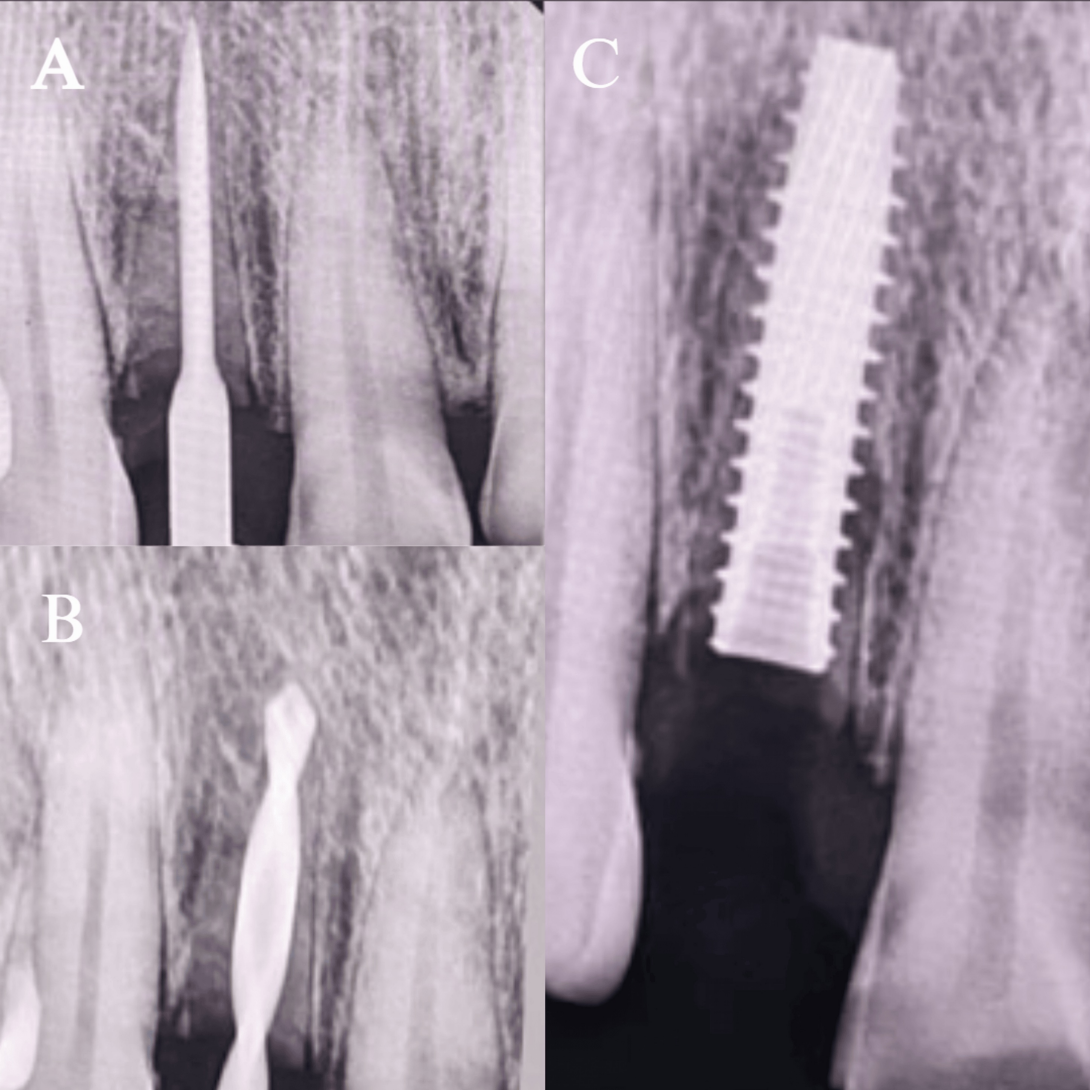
Implant placement in relation to #12 (A) is the radiograph showing the initial placement of the Starter drill; (B) is the radiograph showing the depth of placement with the last osteotomy drill. (C) shows the radiograph post-implant placement
